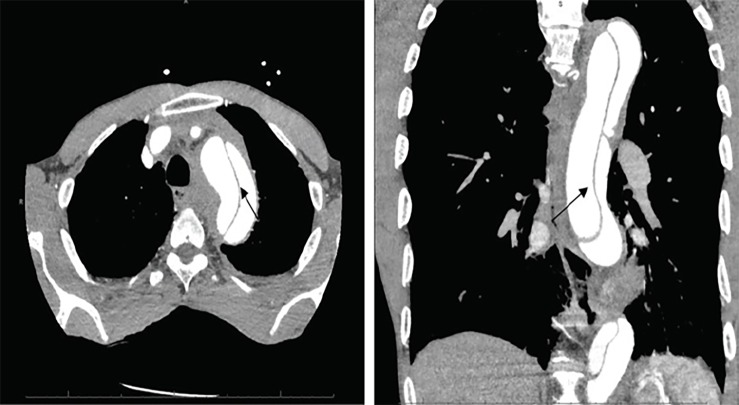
Transverse (left) and coronal (right) computed tomography imaging of the thoracic aorta. Images show a large aortic dissection extending distal from the left subclavian artery. An arrow points to the intimal flap that is visible within the aorta.
